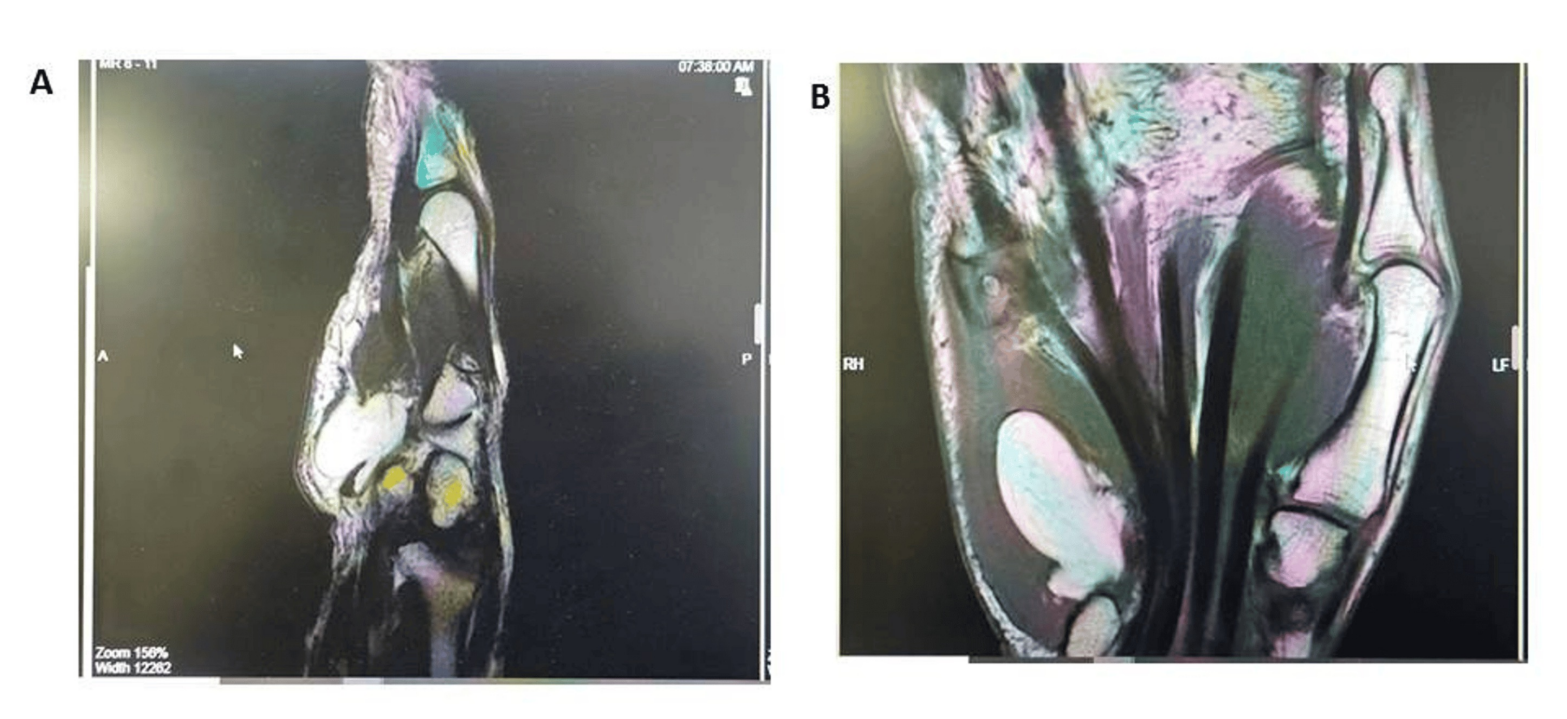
Coronal and sagittal planes of MRI findings of lipomatous mass
